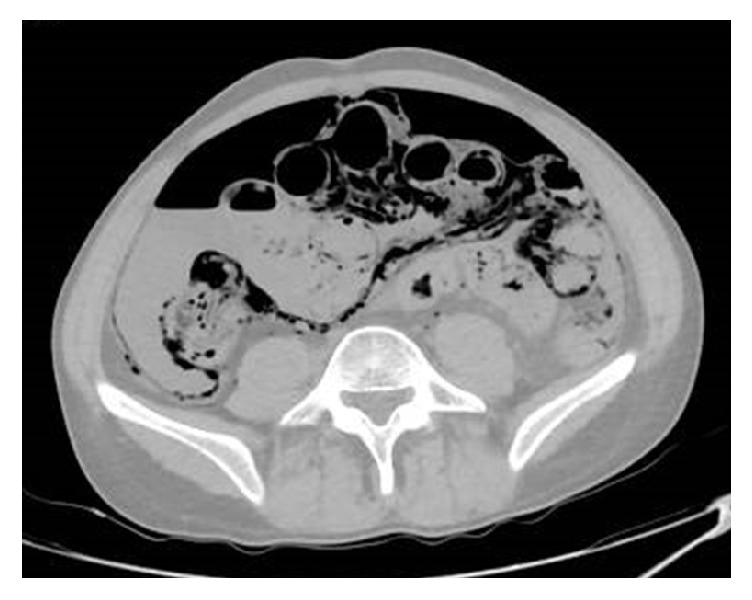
Postmortem CT, axial view, revealing pneumatosis intestinalis along the small bowel and right colon along with free air and unclear fluid in the peritoneal cavity.
